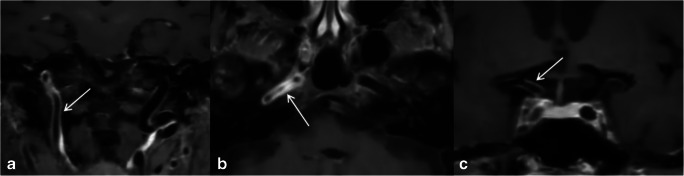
GCA patient with intracranial vasculitic involvement: T1-weighted MRI reveals long-segmental concentric wall thickening and enhancement of the right ICA in its extra- and intradural course (a–c, white arrows)
